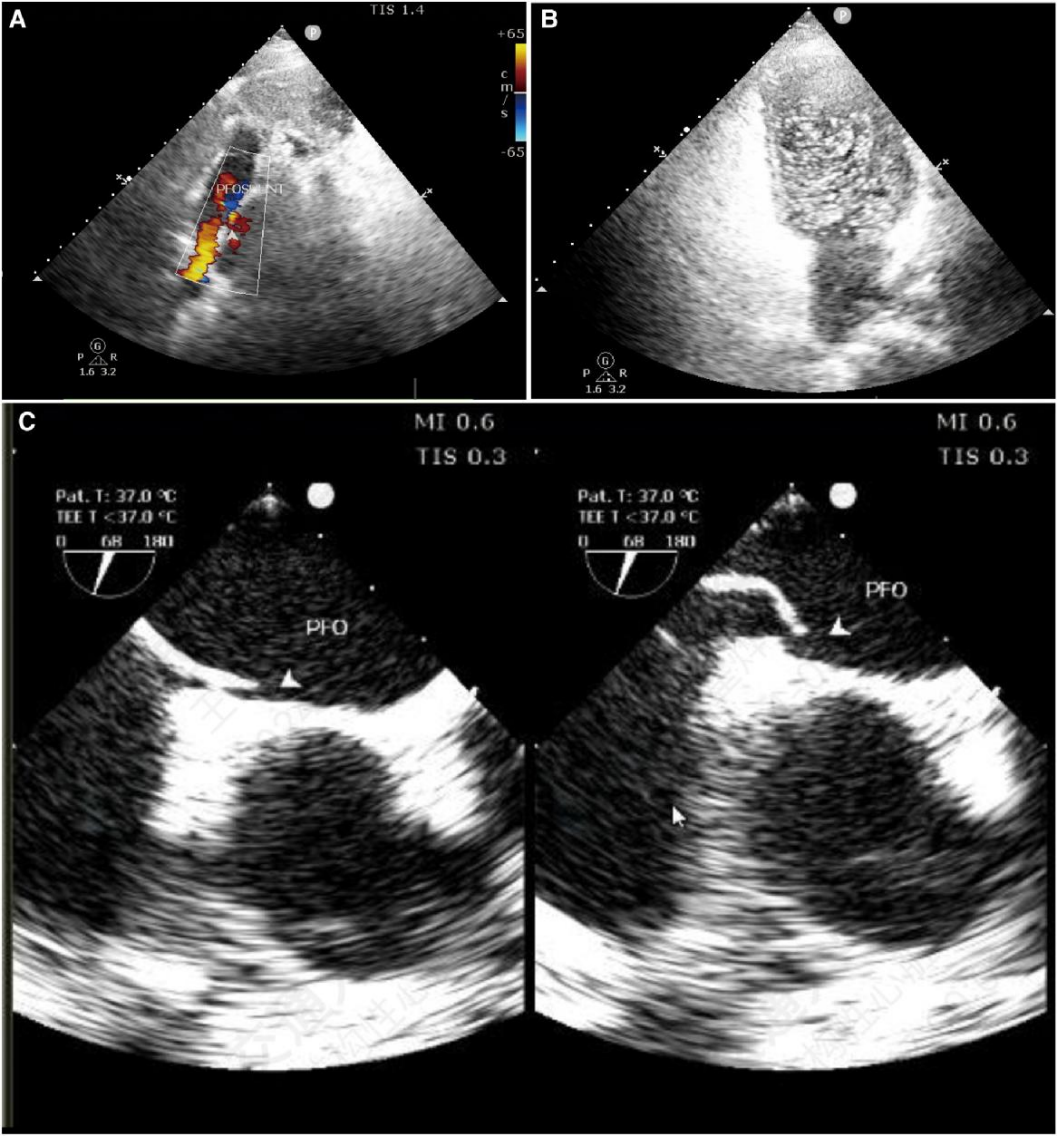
Images of echocardiogram: (*A*) Oblique shunt on atrial septum was revealed by transthoracic echocardiography; (*B*) contrast transthoracic echocardiography shows a large right-to-left shunt; (*C*) anatomy of patent foramen ovale indicated by transoesophageal echocardiography.
